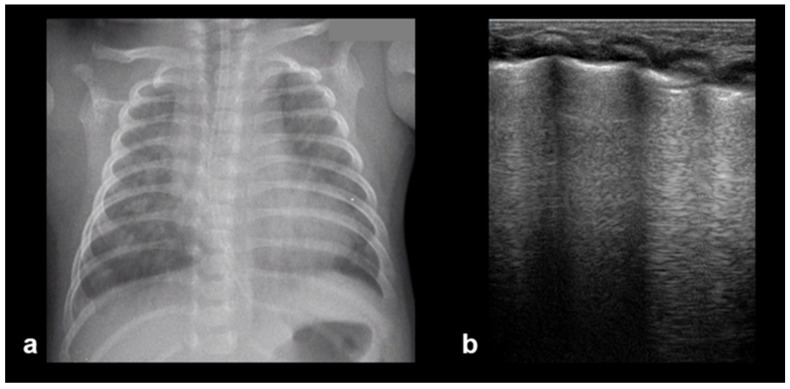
Severe RDS shown on chest X-ray (a); LUS (b). The ability of the LUS to characterize the severity of the RDS is debatable.
